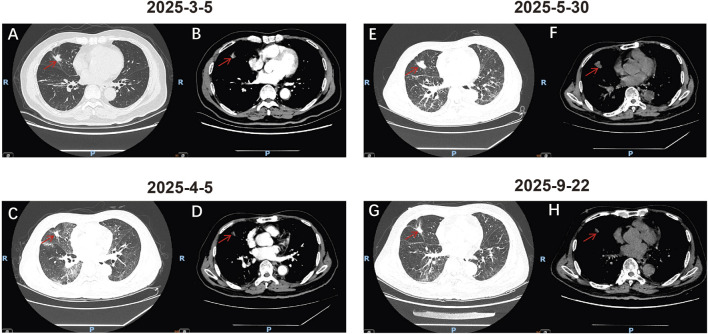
Radiological Assessment of the Primary Tumor. (A,B) Chest CT images on 5 March 2025, showing the primary lesion in the lateral segment of the right middle lobe, measuring approximately 17 × 13 mm after treatment for bone metastases. (A) Lung window. (B) Mediastinal window. (C,D) Chest CT images on 5 April 2025, showing a slight reduction in the size of the primary lesion to approximately 15 × 10 mm. (C) Lung window. (D) Mediastinal window. (E,F) Chest CT images on 30 May 2025, demonstrating an increase in the size of the primary lesion to approximately 24 × 16 mm. (E) Lung window. (F) Mediastinal window. (G,H) Chest CT images on 22 September 2025, showing a reduction in the size of the primary lesion to approximately 16 × 13 mm following aumolertinib rechallenge. (G) Lung window. (H) Mediastinal window.
